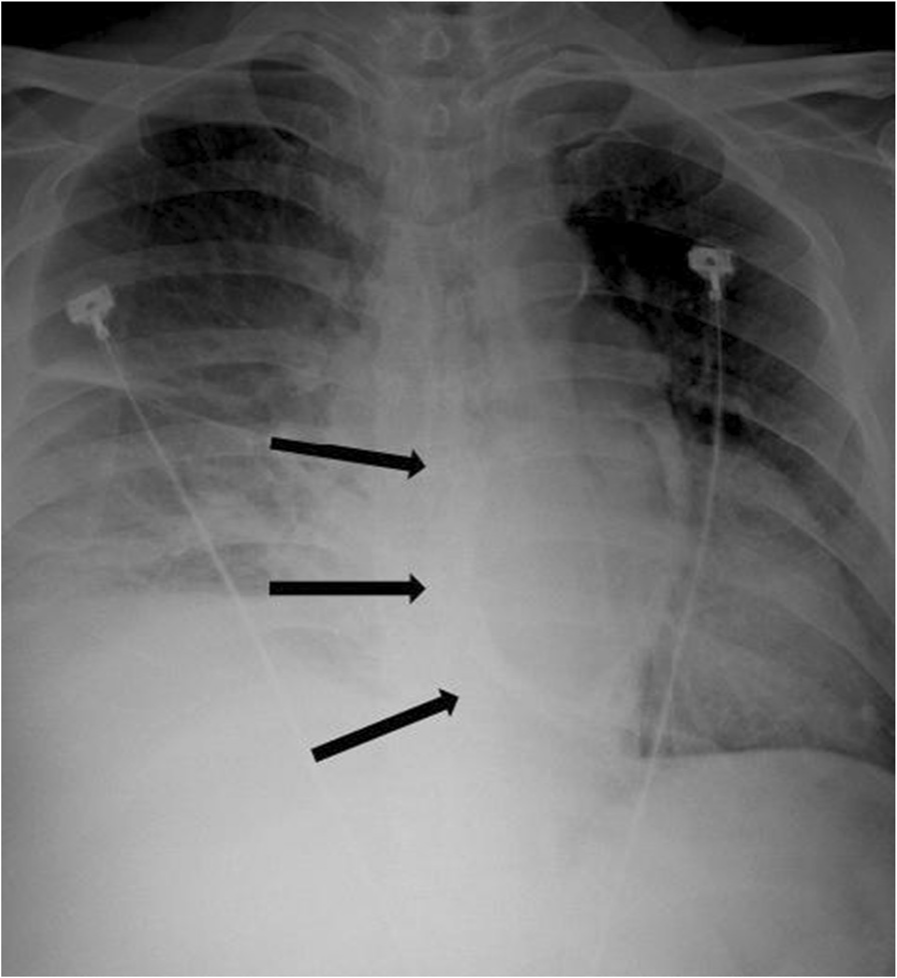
A chest roentgenogram showed a ring-shaped pericardial calcification (arrows).
